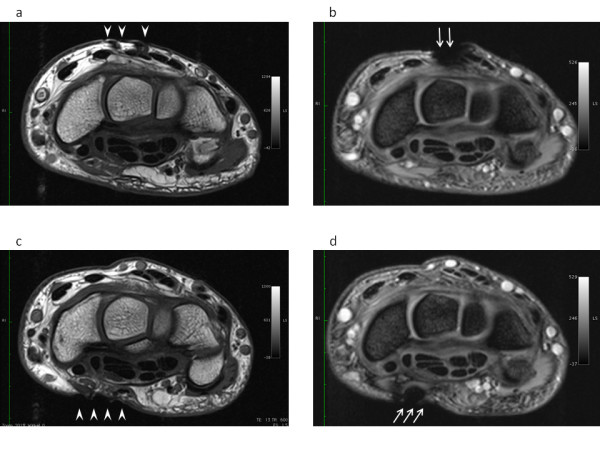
Qualitative image artifacts. MR imaging findings in a volunteer with the RFID tags positioned on the dorsum of the wrist (a, b) and on the volar aspect of the wrist (c, d). Axial T1-weighted spin-echo MR-image (600/13; number of signals acquired, 1; field of view, 90 mm) shows only minimal geometric distorsion and susceptibility artifacts on skin and underlying subcutaneous tissue (arrowheads) (a and c). Axial T2-weighted Flash2D gradient-echo MR-image (400/15; number of signals acquired, 2; field of view, 90 mm) shows increased susceptibility artifacts on skin and underlying subcutaneous tissue and tendons (arrows) (b and d). Interpretation of articular structures is not compromised.
